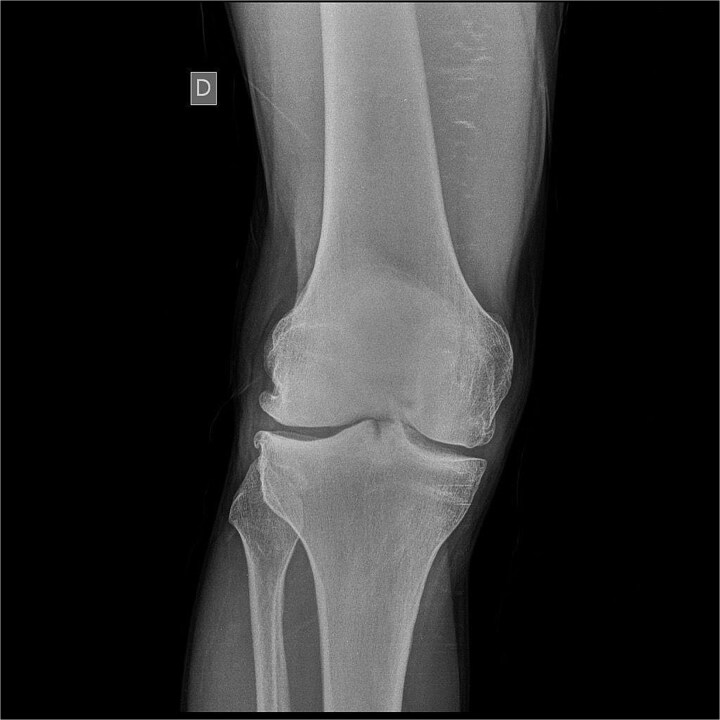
Frontal X-ray of the right knee showing joint space narrowing, osteophytes, and signs of subchondral sclerosis.
